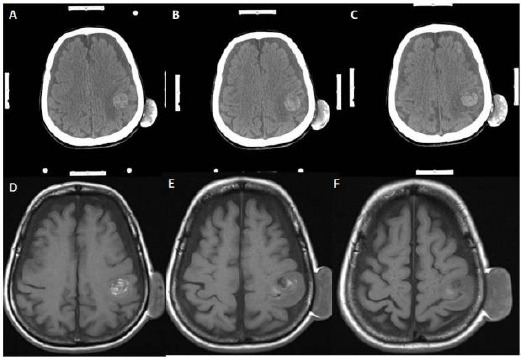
A, B, C (Table 1: Case 2, Cavernoma): Noncontrasted computerized cranial tomography showing that hyperdense oval shaped lesion having hypodense areas within it. D, E; F: Noncontrasted T1 weighted axial cranial magnetic resonance image scans showing the heterogenous intensity and edematous appearance at the left motor cortex. Also, trichilemmal cyst is shown at the scalp these scans*.
